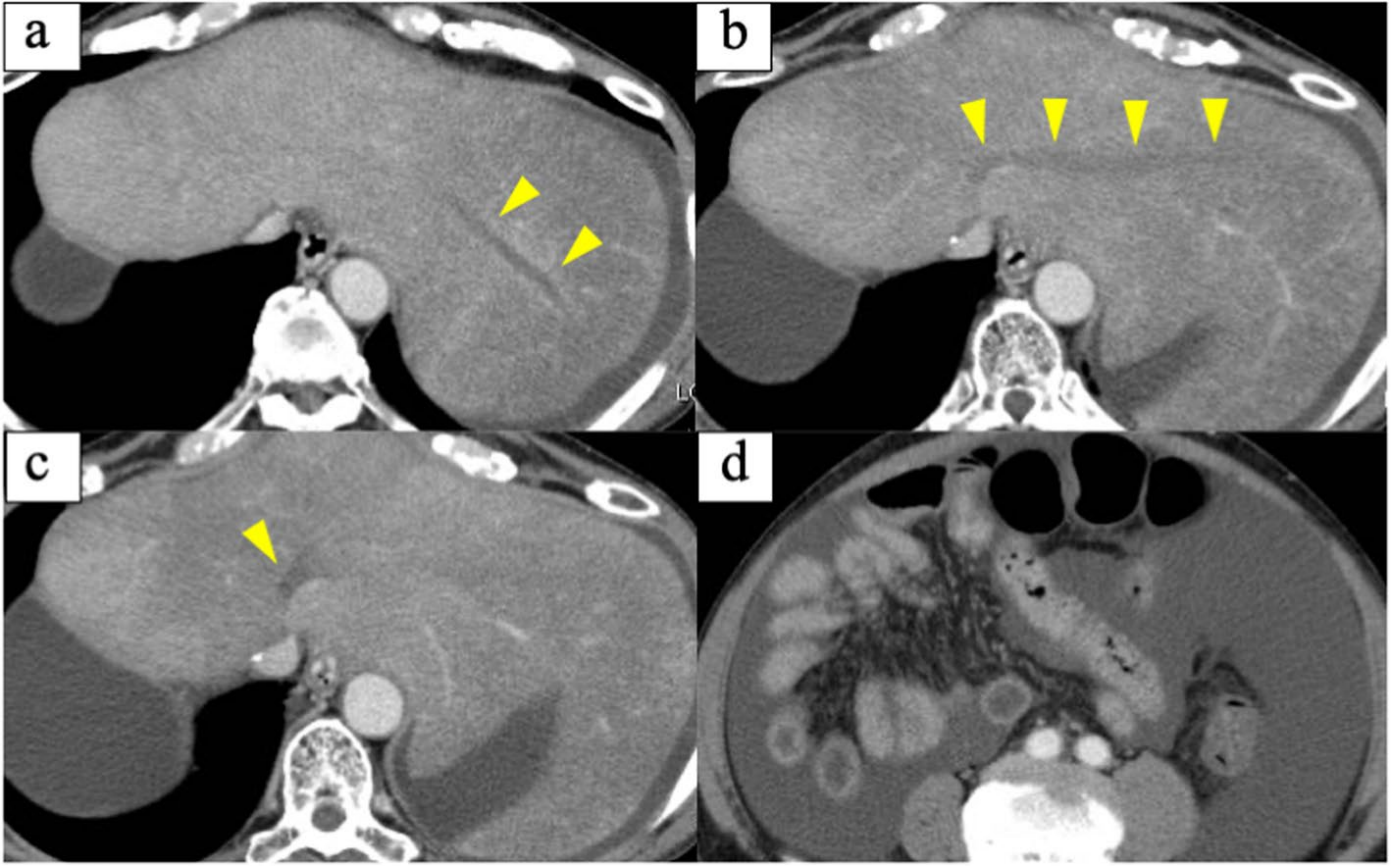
Multidetector-row computed tomography findings at the 32nd postoperative month. (before treatment for Budd-Chiari syndrome). a, b, c Yellow arrowheads show thrombotic occlusion of the LHV. d Massive ascites was confirmed
